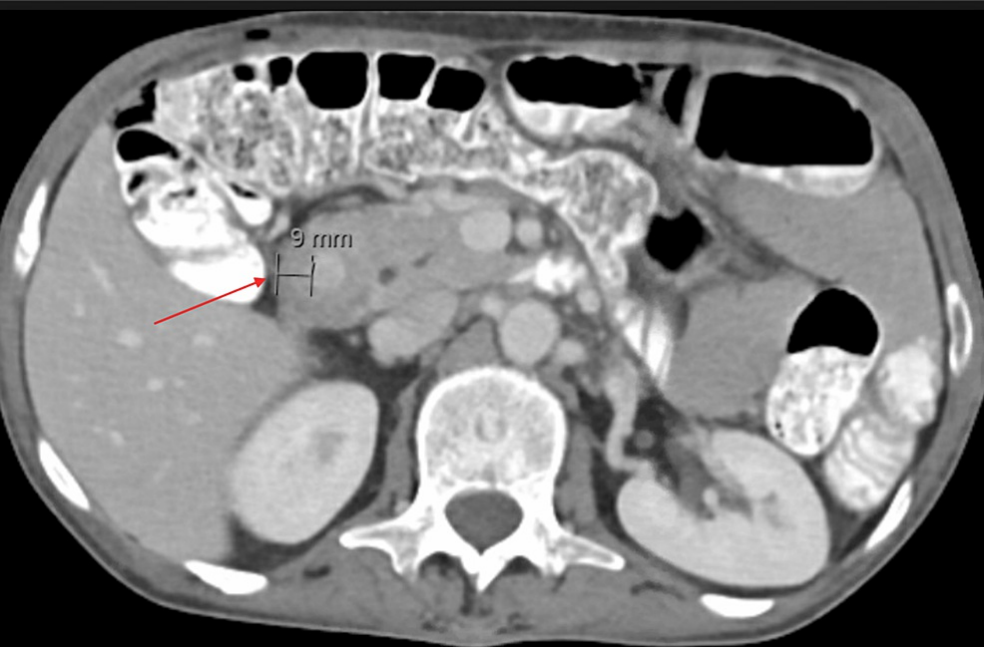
CT with contrast. A 9 mm thickening of the second part of the duodenum. CT = computerized tomography
